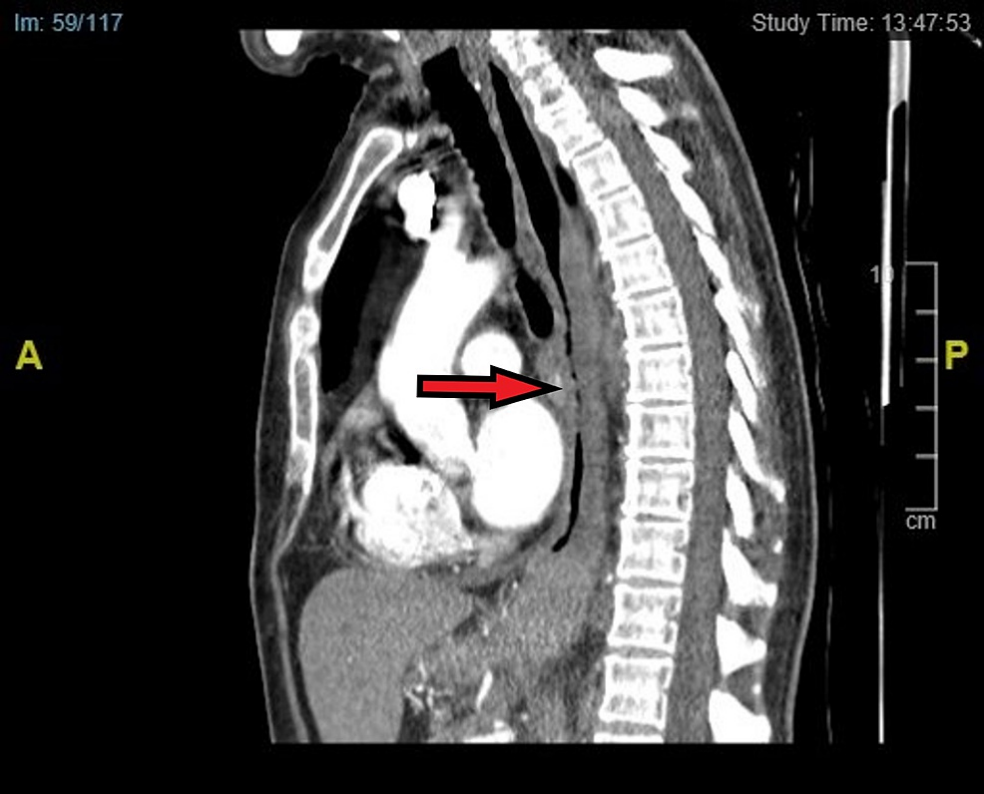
Sagittal plane view of our patient’s CT thorax showing stricturing oesophagus from middle to distal section This correlates with our OGD finding as described in Figure 1.
